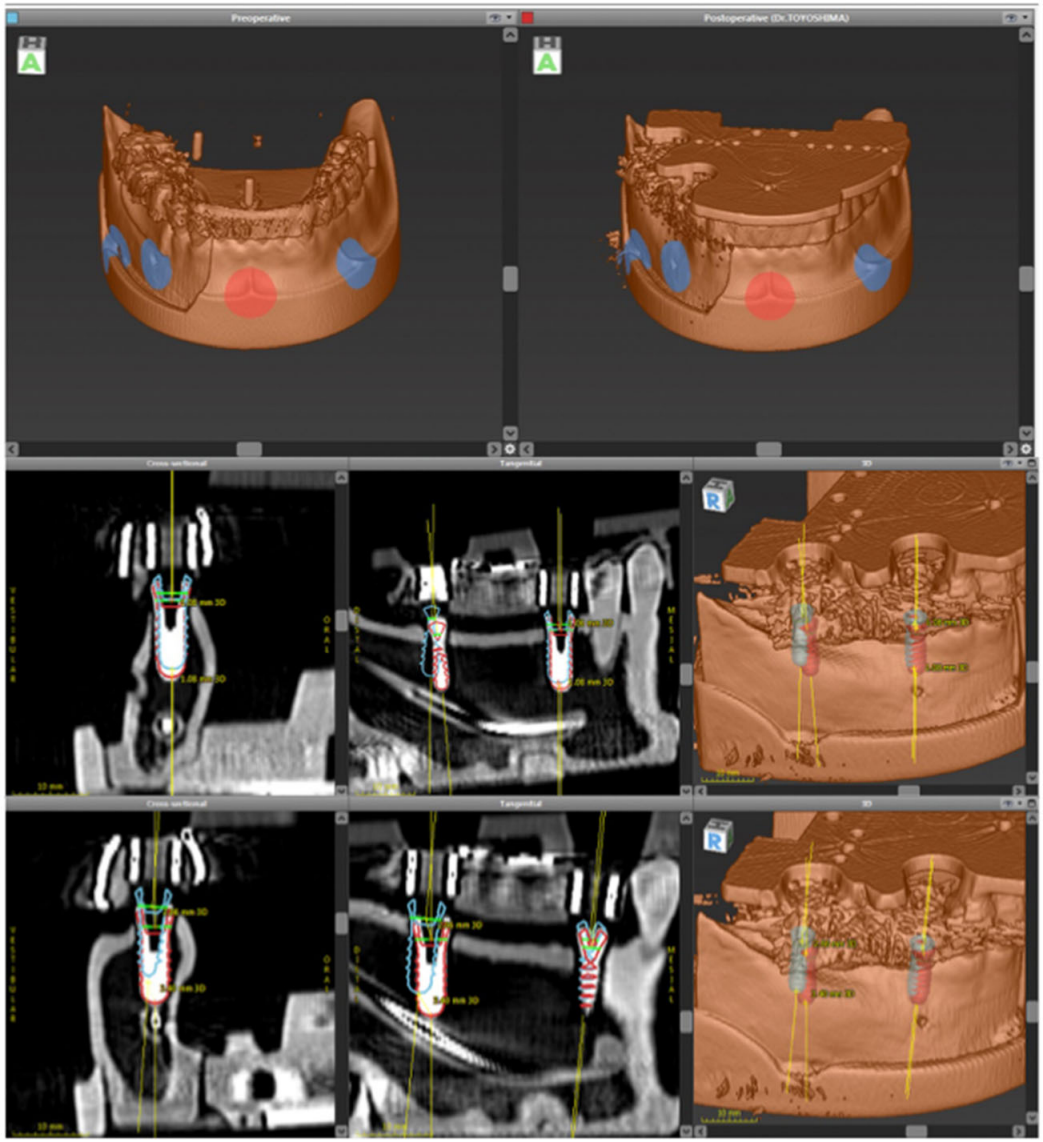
Accuracy evaluations of implants. Postoperative computed tomographies were performed in compliance with the preoperative image acquisition and transformed into the digital imaging and communications in medicine format. The preoperative planning datasets were manually aligned with the postoperative data using rigid registration and anatomical landmarks.
